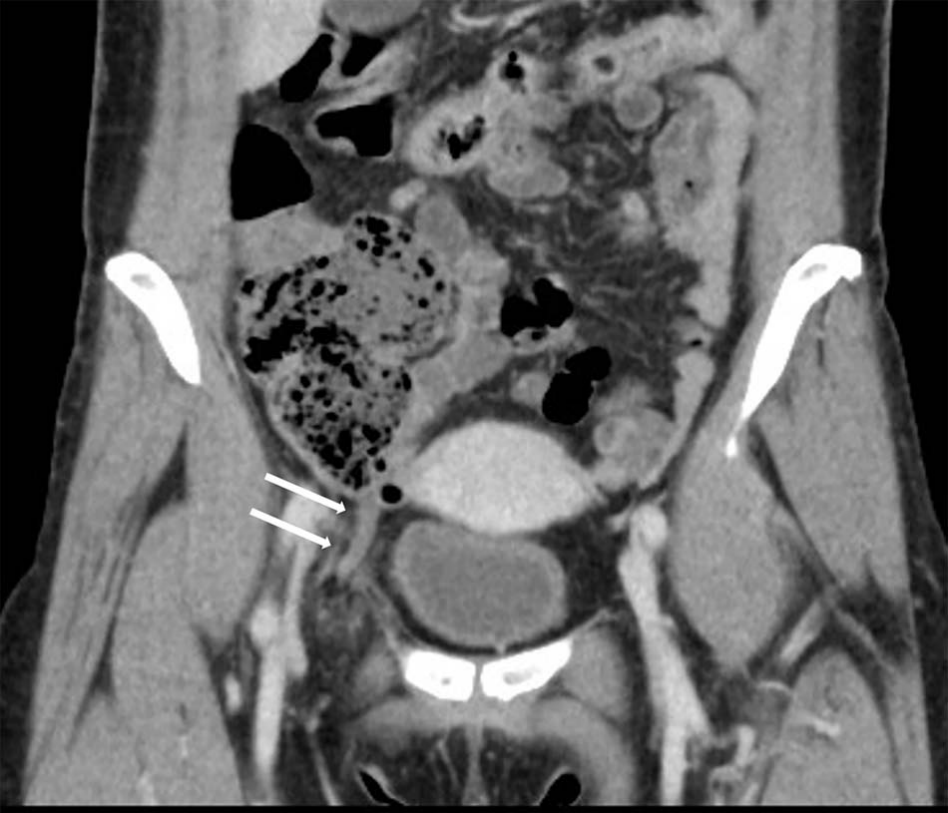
Coronal view in the abdominal CT scan shows the appendix inside the right femoral canal.
